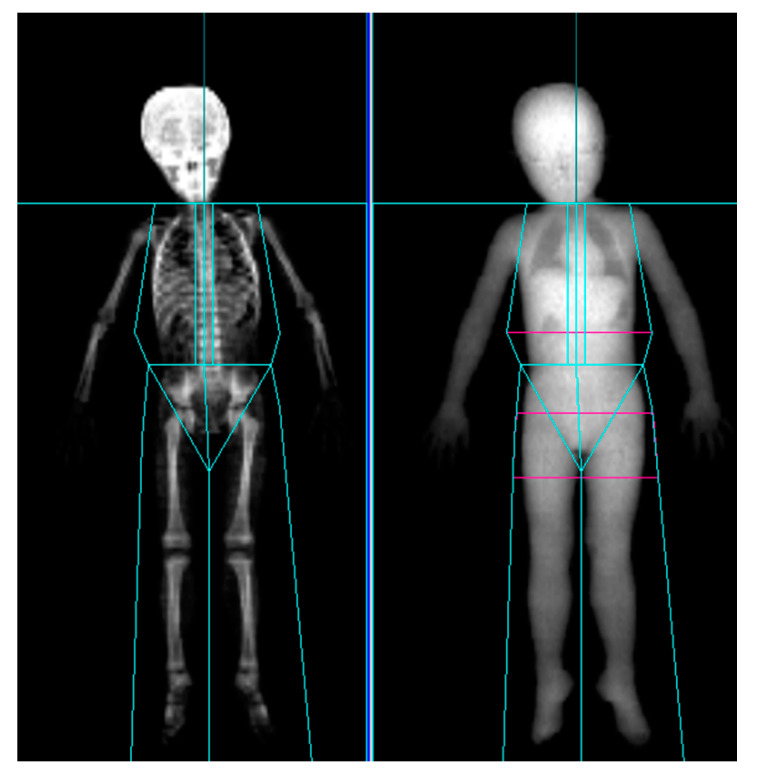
Dual-energy X-ray absorptiometry scan of a 4-year-old cerebral palsy patient showing positions of regional markers.
